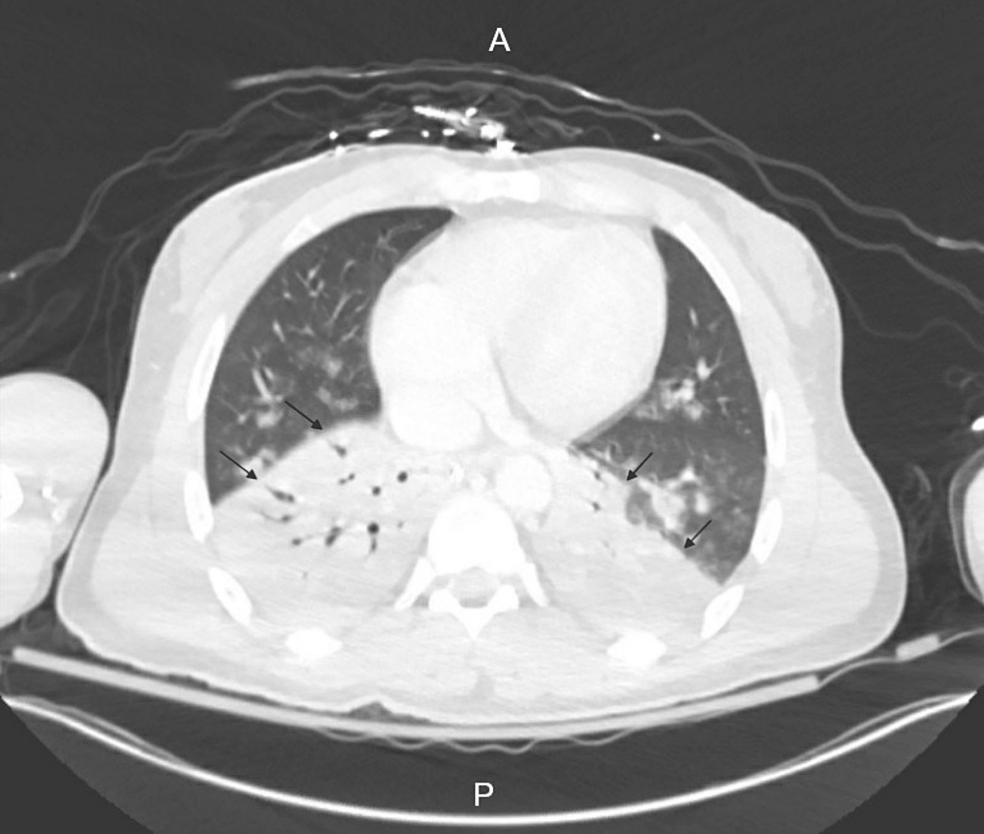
Chest computed tomography scan showing multilobar pneumonia, with greatest consolidation in lower lung lobes (black arrows) A, anterior; P, posterior
